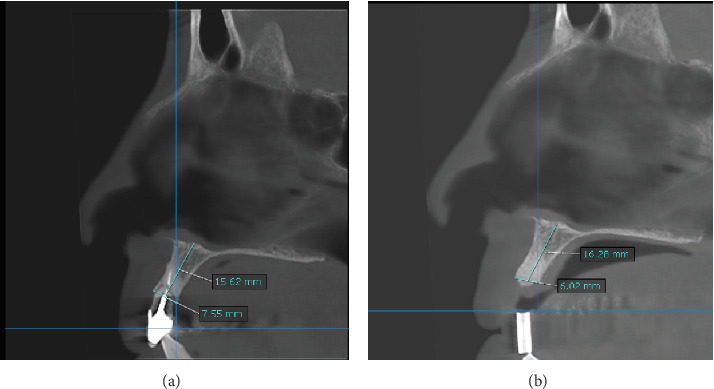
(a) Baseline measure CBCT of the upper central incisor (Bartee technique). (b) Measure of 6 months of healing.
